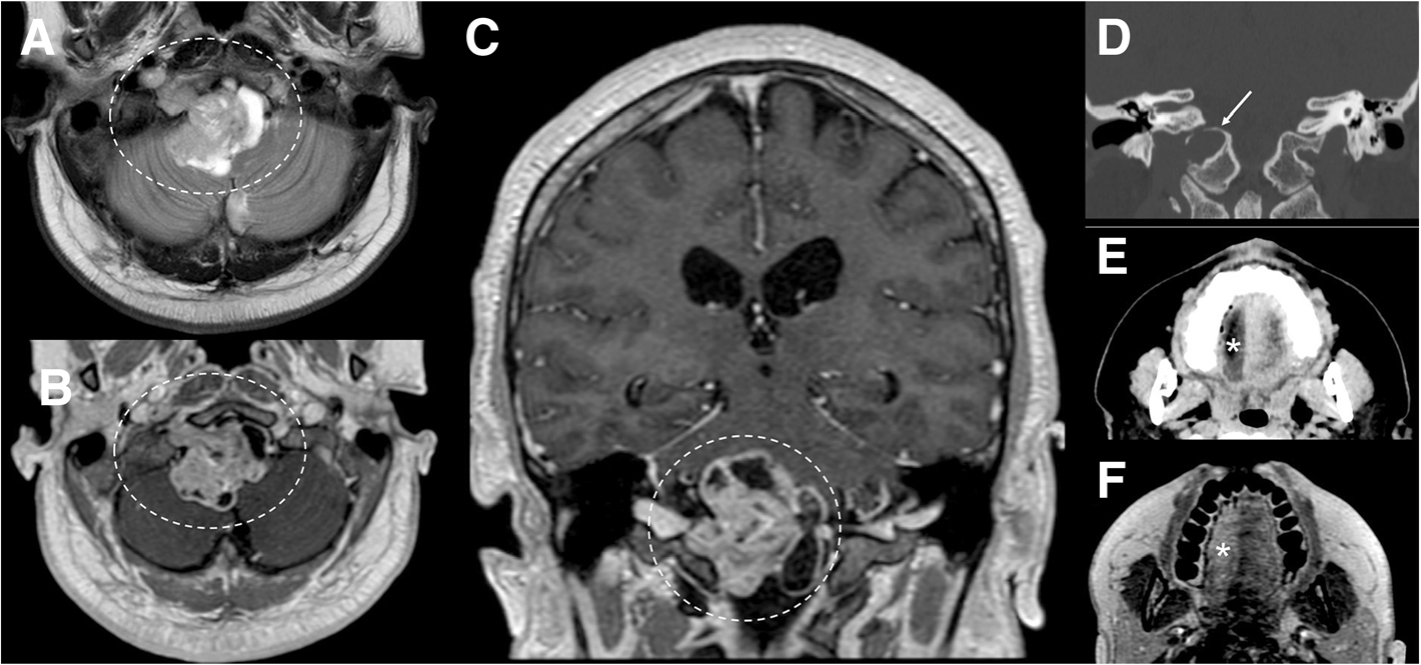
Hypoglossal schwannoma. MRI T2-weighted axial image (a), T1-weighted post-gadolinium axial (b), and coronal images (c) demonstrate an extra-axial expansive lesion (dotted circles), mildly hyperintense with heterogenous contrast enhancement, surrounded by cystic components. The mass is located along the course of the right hypoglossal nerve. CT (bone window, d) clearly shows the enlargement of the right hypoglossal canal (arrow). Atrophy of the right tongue muscles (asterisks) is well visible at CT (e) and T1-weighted axial sequence (f) as hypodense and hyperintense area, respectively
